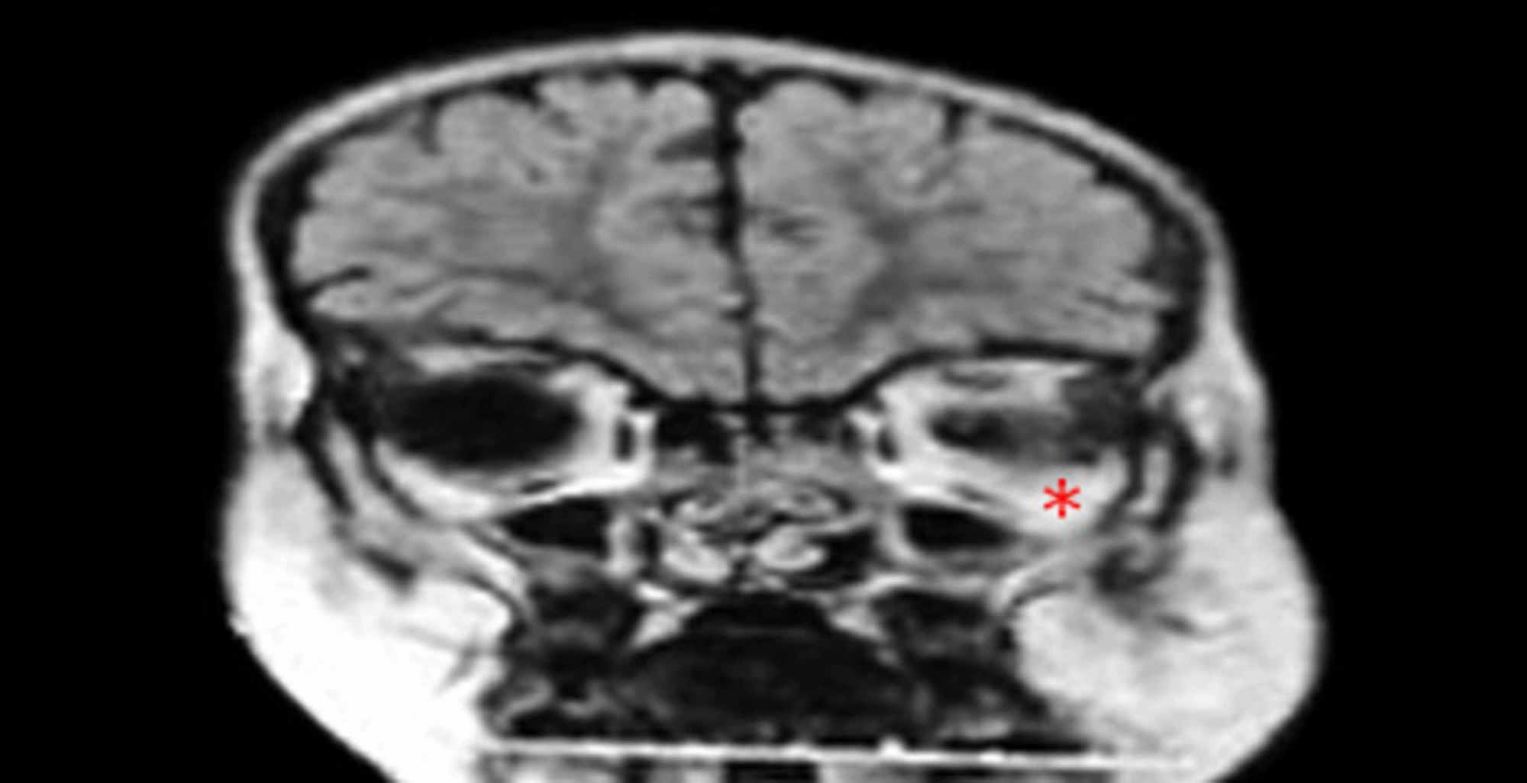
Magnetic resonance imaging (MRI) coronal view showing a well-defined abnormal single intensity lesion in the left temporal and left masticator space involving the medial pterigoid, lateral pterigyoid, masseter, and temporalis muscles; it also involves the left maxilla, infratemporal area, left parapharyngeal space, and left oropharynx area *= Tumor
